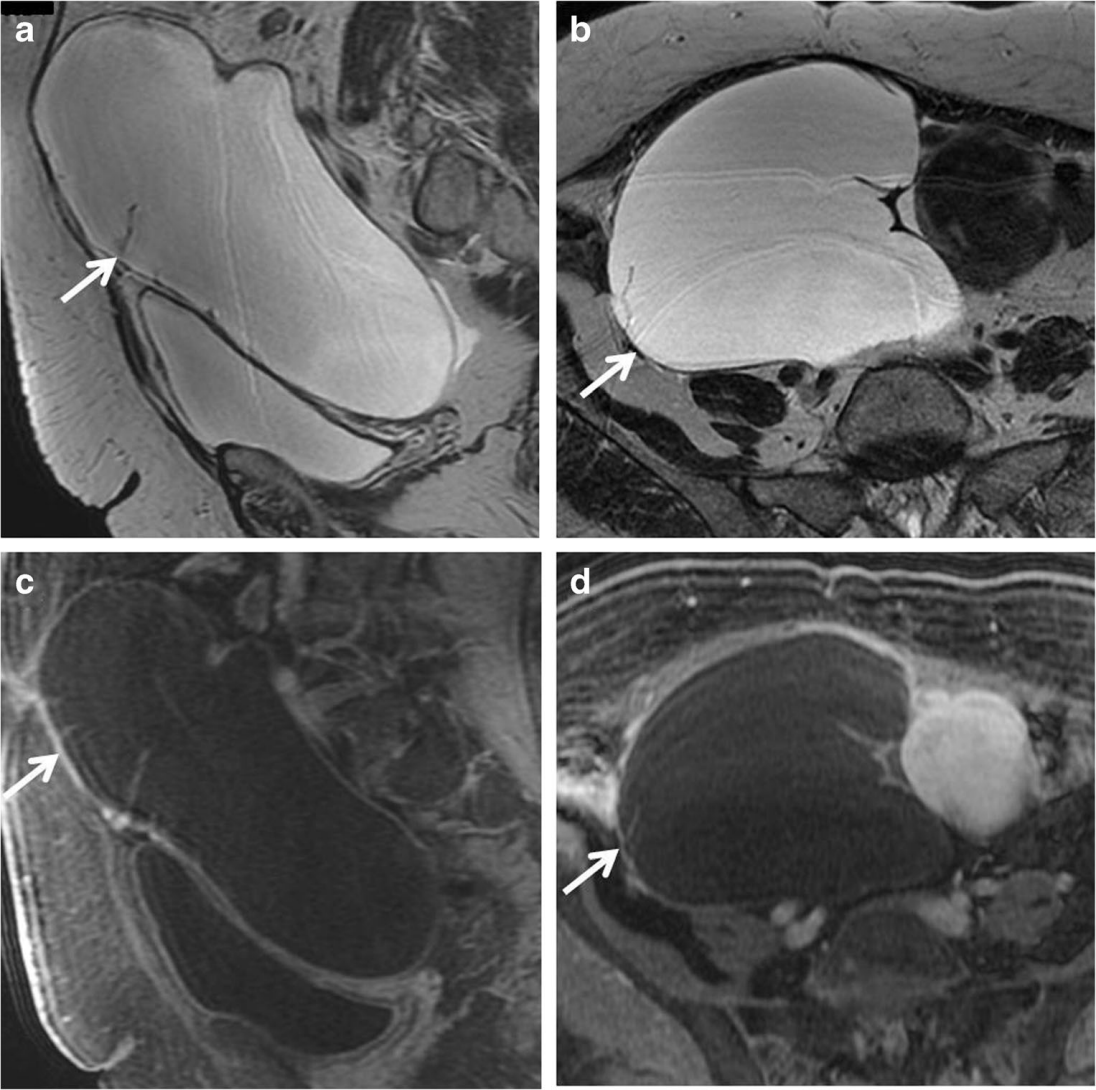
Serous cystadenoma in a 64-year-old woman. (a) Sagittal and (b) axial T2-weighted images show a hyperintense unilocular cystic mass (*white arrows*). On (c) sagittal and (d) axial contrast-enhanced fat-suppressed T1-weighted images, the cyst wall shows poor contrast enhancement (*white arrows*) without vegetations, nodularity, or solid components
